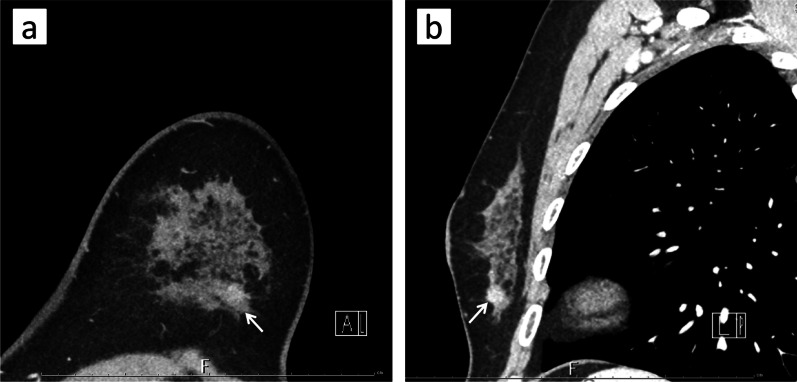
Enhanced computed tomography. Solitary heterogeneously enhanced mass at the outer lower area of the left breast
